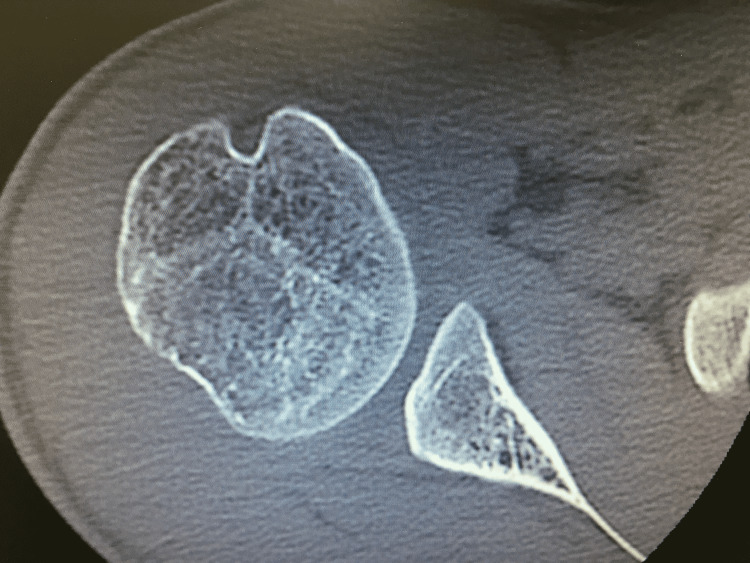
CT scan showing glenoid dysplasia with a loss of posteroinferior concavity with 28 degrees of retroversion
